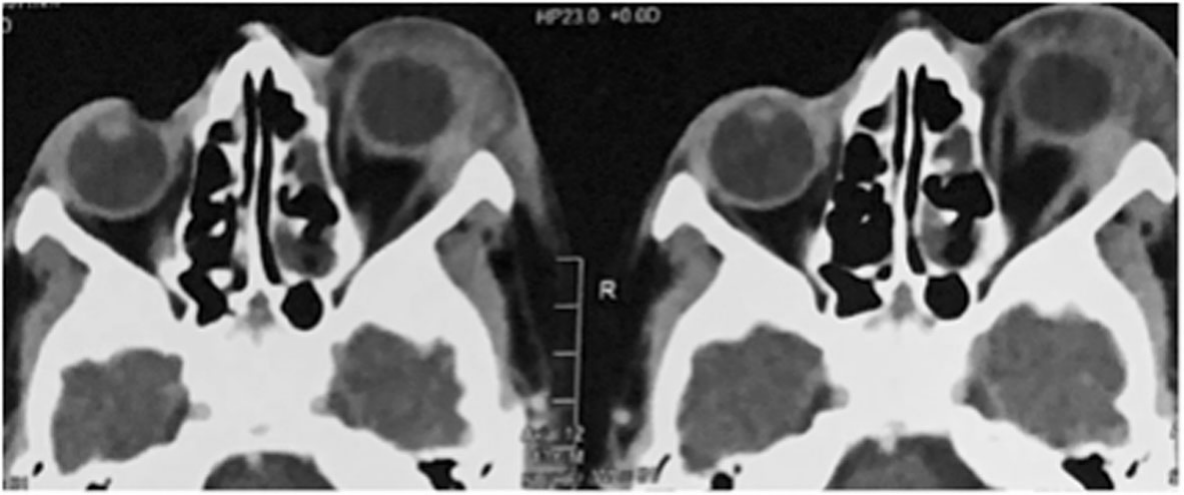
Axial orbital CT scan showing left orbital cellulitis with axial proptosis
